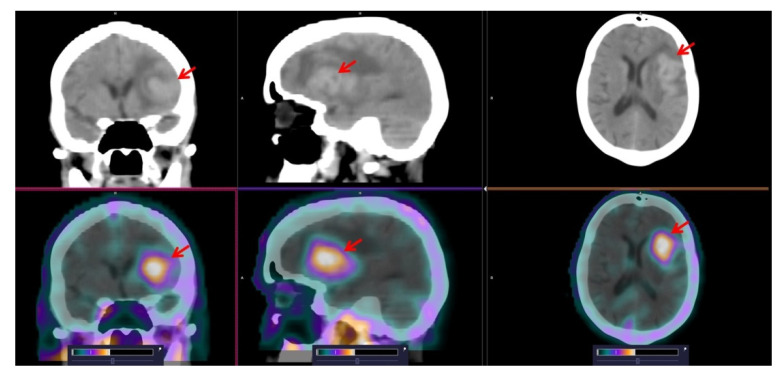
SPECT/CT images of patient 12 at 2 h after injection of [^99m^Tc]Tc-TG. High [^99m^Tc]Tc-TG uptake in left frontoparietal region of the brain (SUVmax = 2.65) is visualized (arrows). The upper setting of the scale window (65% of the maximum number) was adjusted to visualize the lesion.
